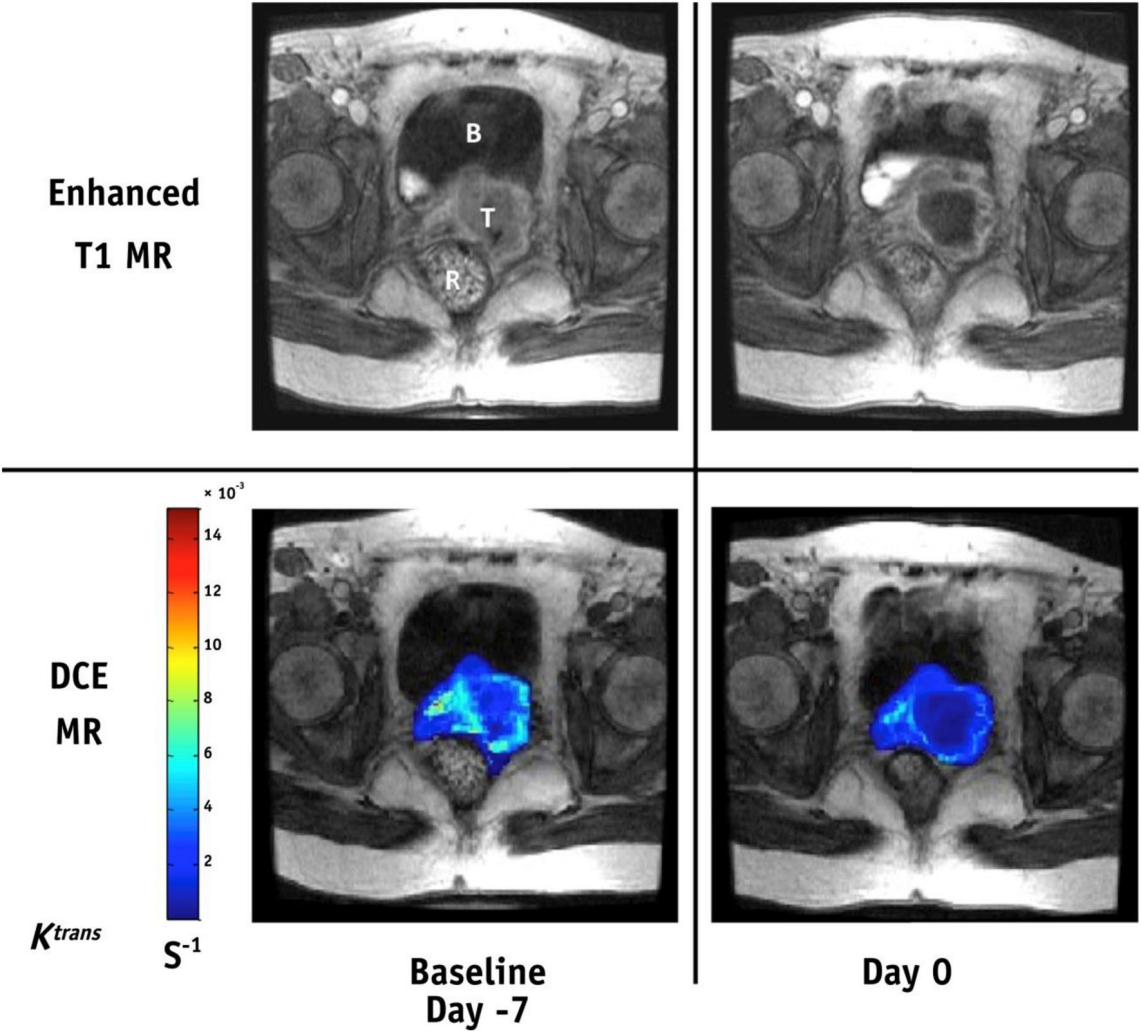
Changes in cervix tumor hypoxia by the angiogenesis inhibitor sorafenib, assessed by DCE-MRI parameter K^trans^. Late-phase axial DCE-MR T1 image of pelvis (*upper*) and the corresponding tumor K^trans^ maps (*lower*) superimposed on the late-phase DCE-MR T1 image at baseline before any treatment (day −7; *left*) and after 7 days of sorafenib alone (day 0; *right*). Note the decrease in K^trans^ after sorafenib treatment. *B* bladder, *R* rectum, *T* tumor Reproduced with permission from Milosevic et al. [8]
